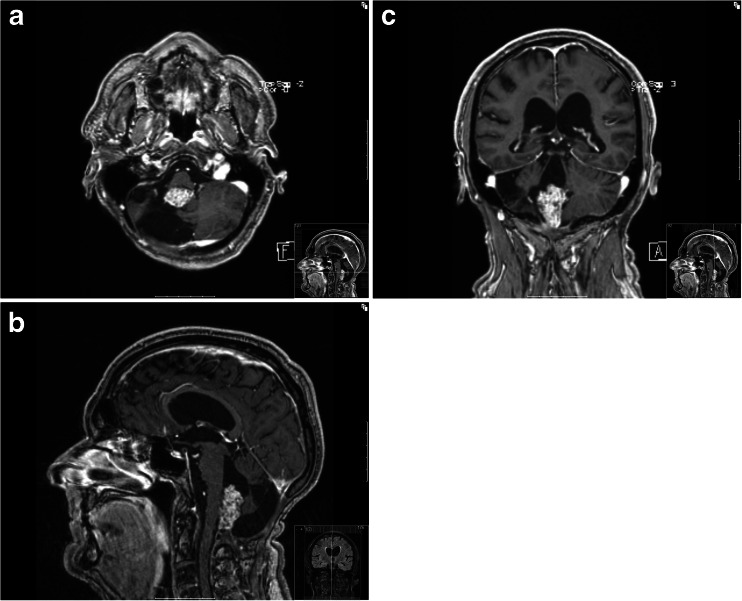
MRI of the posterior fossa discloses contrast-enhancing tumor in the fourth ventricle. The situation has been stable for several years, and the patient is without clinical symptoms and signs
